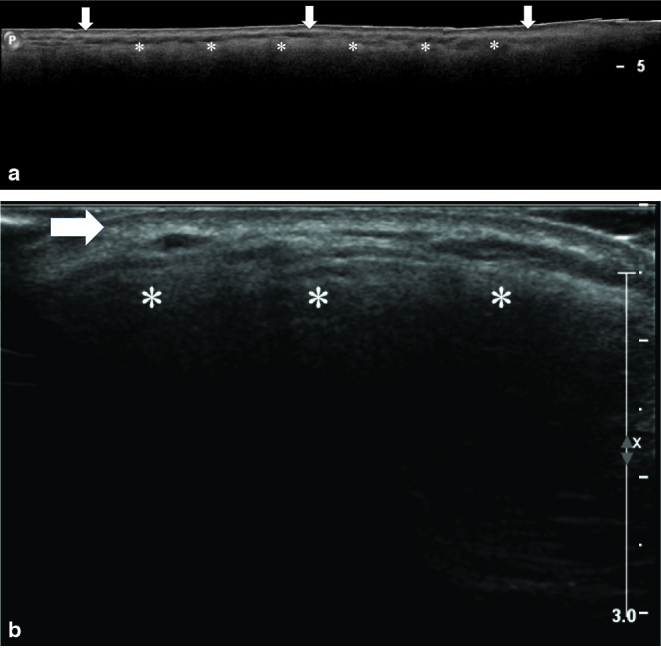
(a) Longitudinal extended-field-of-view ultrasono-graphy scan of the anterolateral aspect of the right leg and (b) transverse ultrasonography scan of the anterolateral aspect of the left leg showing normal appearance of the dermis (arrows) and diffuse hyperechogenicity of the subcutaneous tissues (asterisks) with strong posterior acoustic shadowing masking the deeper structures. This “snowstorm pattern” is consistent with liquid silicone filler.
